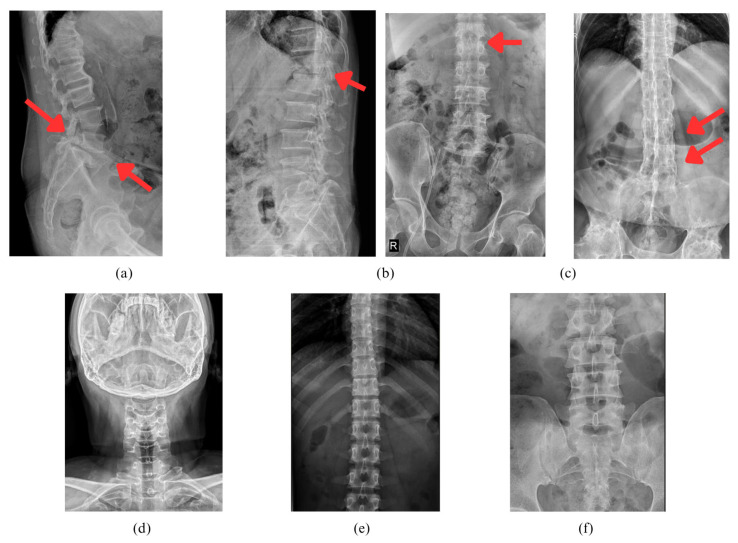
Representative spinal X-ray images for each diagnostic class: (a) spondylolisthesis, (b) infection, (c) spondyloarthropathy (SpA), (d) normal cervical spine, (e) normal thoracic spine, and (f) normal lumbar spine.
